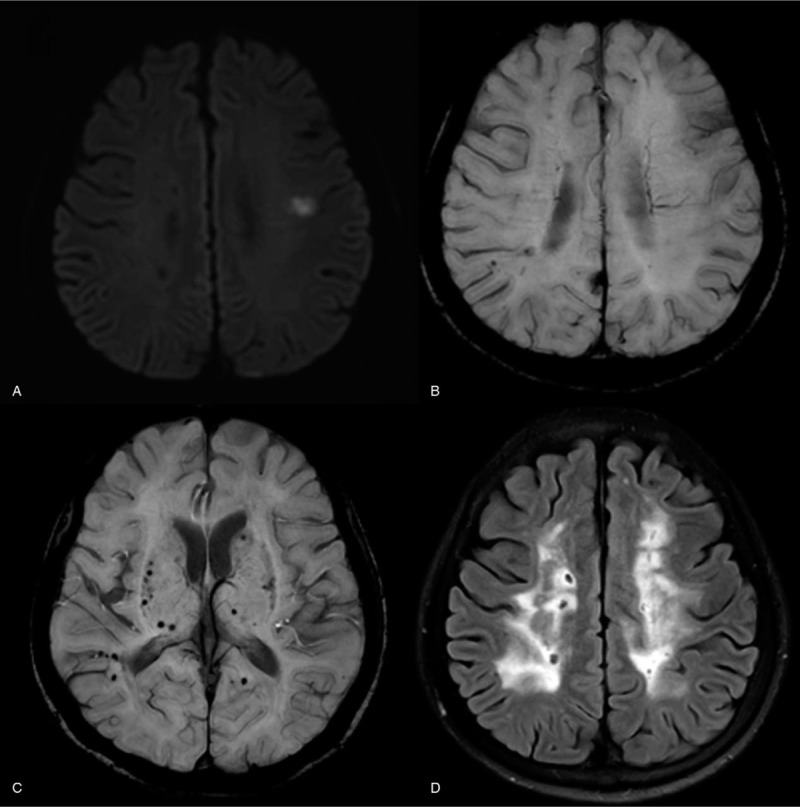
Brain magnetic resonance image obtained 8 months previously. Diffusion-weighted imaging (A) shows increased signal intensity in left corona radiata consistent with acute ischemic stroke. Susceptibility-weighted imaging(B) did not showed any hemorrhage in the ischemic stroke lesion. Susceptibility-weighted imaging reveals multiple micro-bleedings in the temporo-parietal cortex, thalamus and basal ganglia (C), and confluent white matter changes with multiple silent infarctions can be seen in the fluid-attenuated inversion recovery image (D), which implies underlying small vessel disease.
